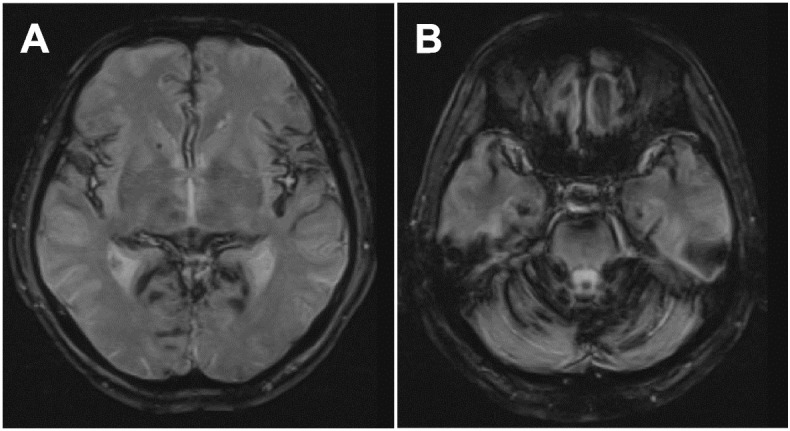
Brain MRI findings. Diffuse and prominent superficial deposition of hemosiderin in the brain on T2*-weighted imaging (A, B)
